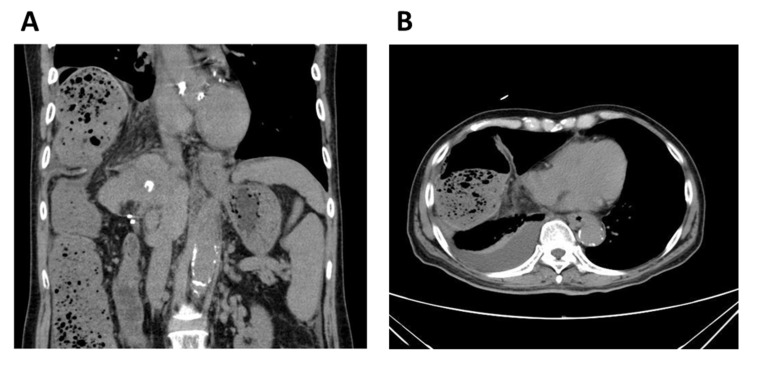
(A) Coronal and (B) axial views of contrast-enhanced computed tomography reveals a right diaphragmatic hernia of the colon with numerous stools.
